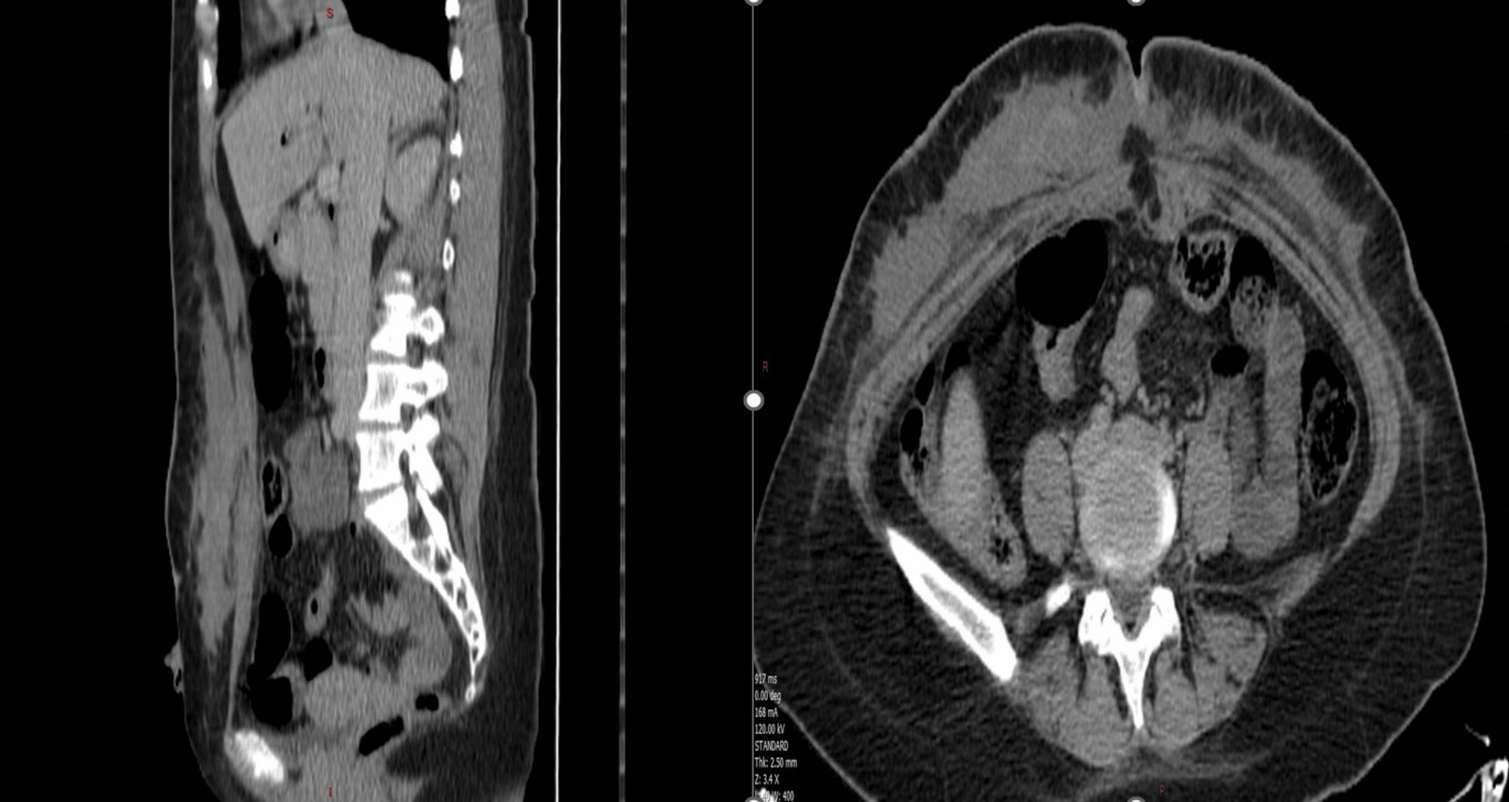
Abdominopelvic CT scan showing extended hematoma and signs of necrotizing fasciitis.
